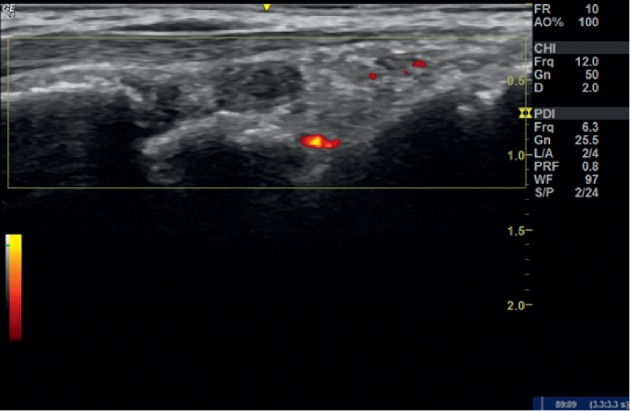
Ultrasound examination of the right wrist at 6 weeks in case 1. Longitudinal view of showing a power Doppler grade 1 synovitis.
